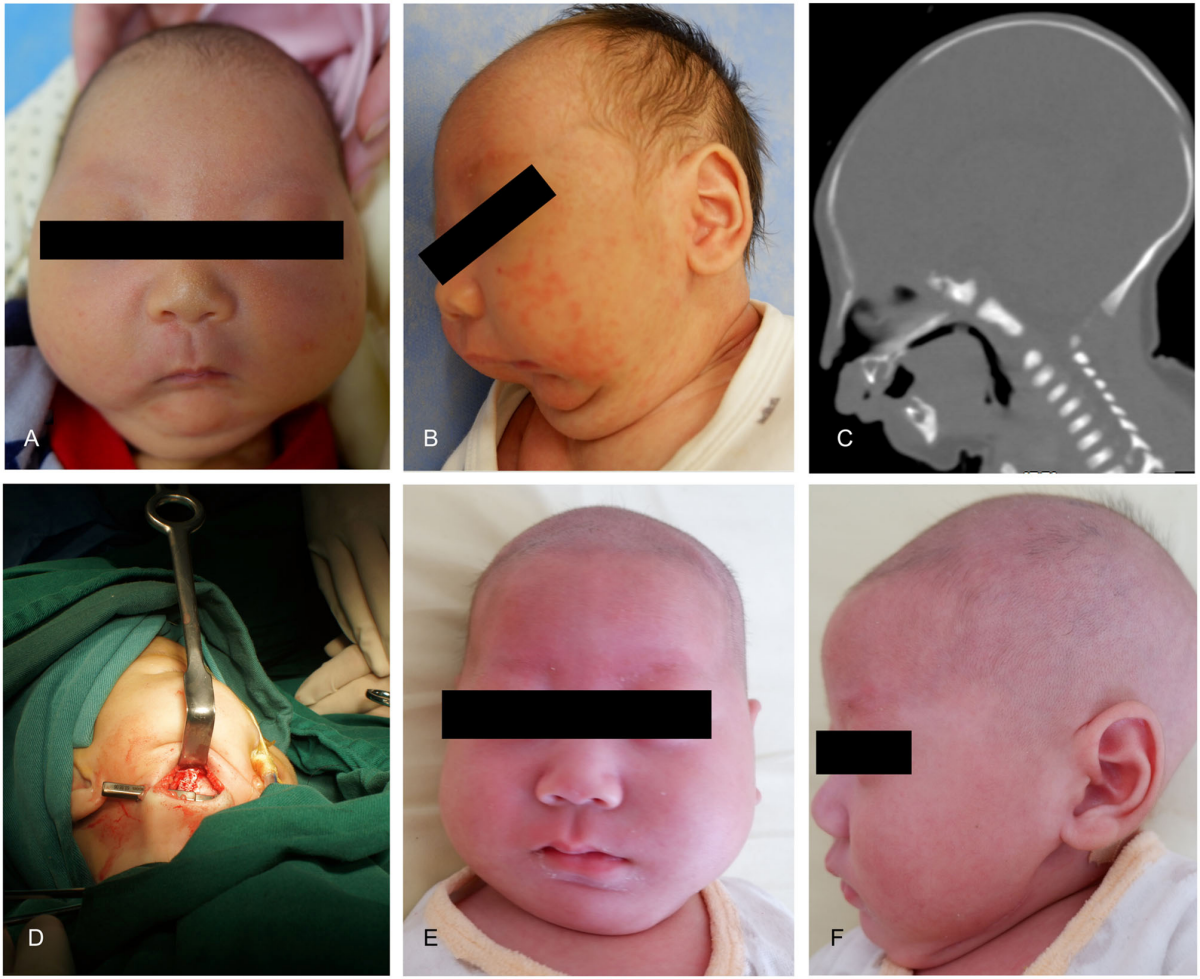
Case 2. (A) A preoperative frontal view. (B) Preoperative lateral view. (C) Preoperative CT-scan. (D) Intraoperative situation. (E) Postoperative anteroposterior view. (F) Postoperative lateral view.
